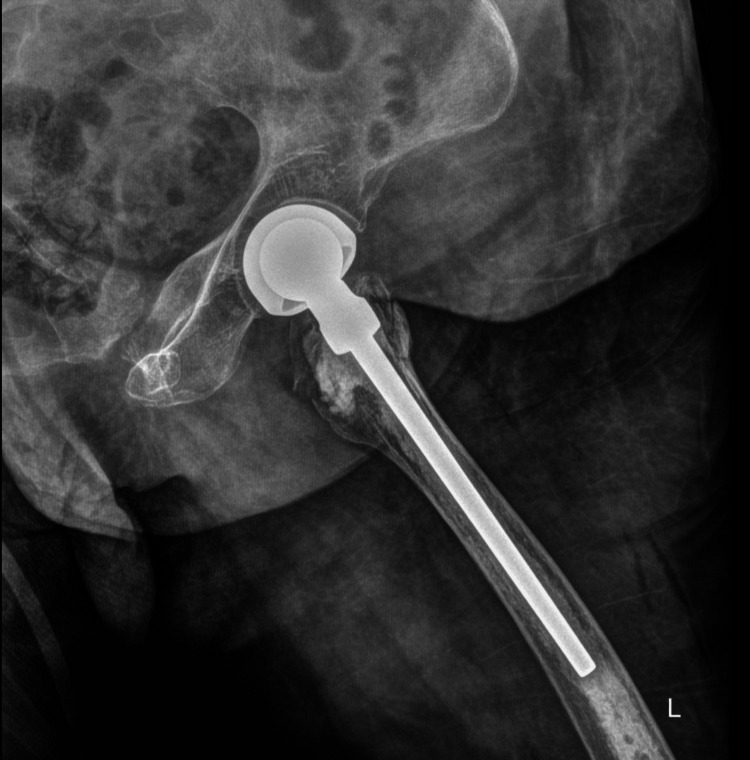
Postoperative radiograph showing proper alignment and positioning of the left hip implant
